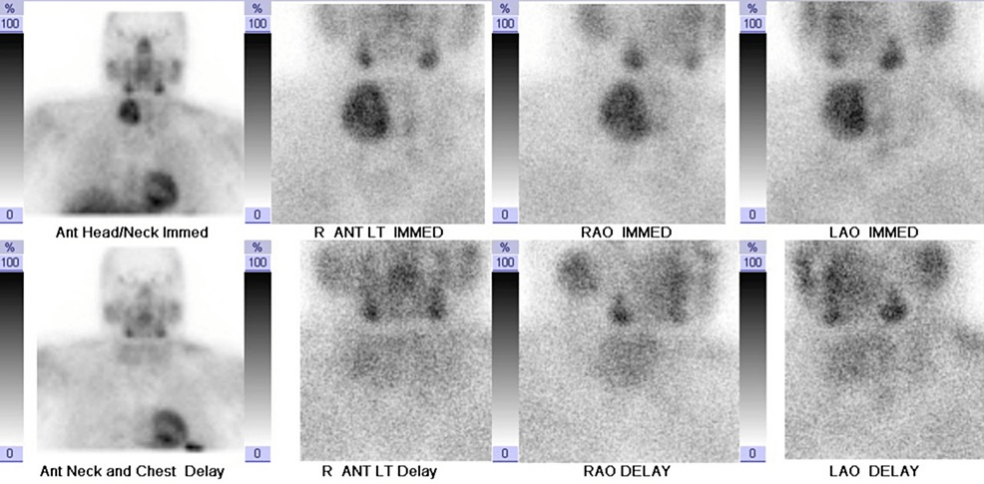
(Upper row) Planar initial 15-minute image and (lower row) 2.5-hour delayed planar image from first nuclear medicine parathyroid scintigraphy after injection of 24.5 millicuries of technetium-labeled sestamibi. The initial image demonstrates asymmetrical increased tracer uptake in the right thyroid region. The delayed image does not show abnormal tracer retention to suggest parathyroid adenoma.
